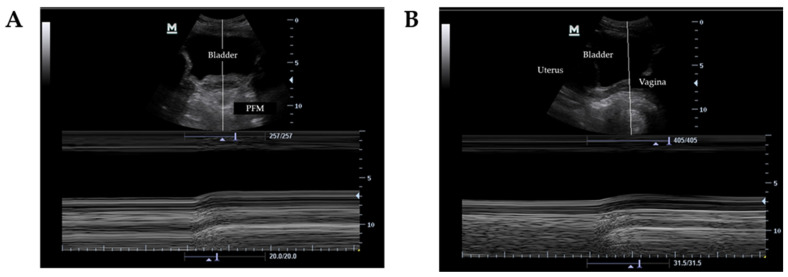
Ultrasound of the bladder base displacement in motion mode M. (A) MVC of PFM in transversal plane. (B) MVC of PFM in sagittal plane.
